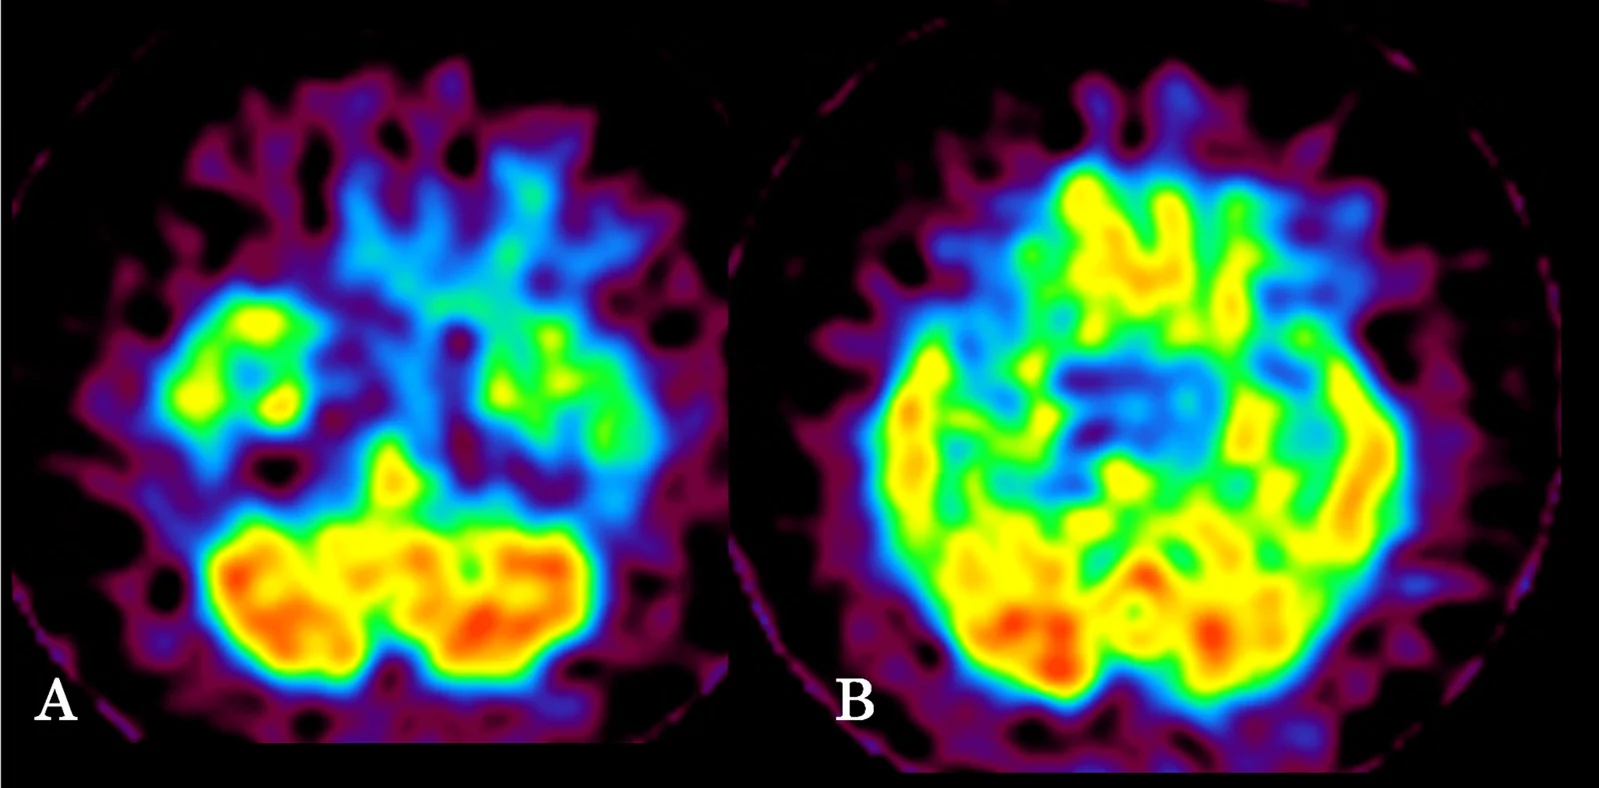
Single-photon emission computed tomography (SPECT) findings SPECT images at (A) the level of the paranasal sinuses and (B) the level of the temporal region. These images show no significant tracer accumulation corresponding to the tumor in the left sphenoid region and no apparent perfusion abnormalities in the surrounding brain parenchyma.
